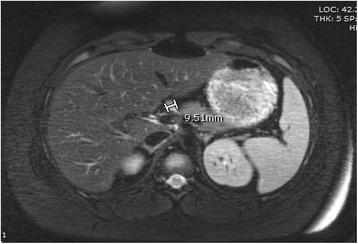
Axial T2 MRI image of the abdomen. The bar represents the 9.5 mm mass in the neck of the pancreas
